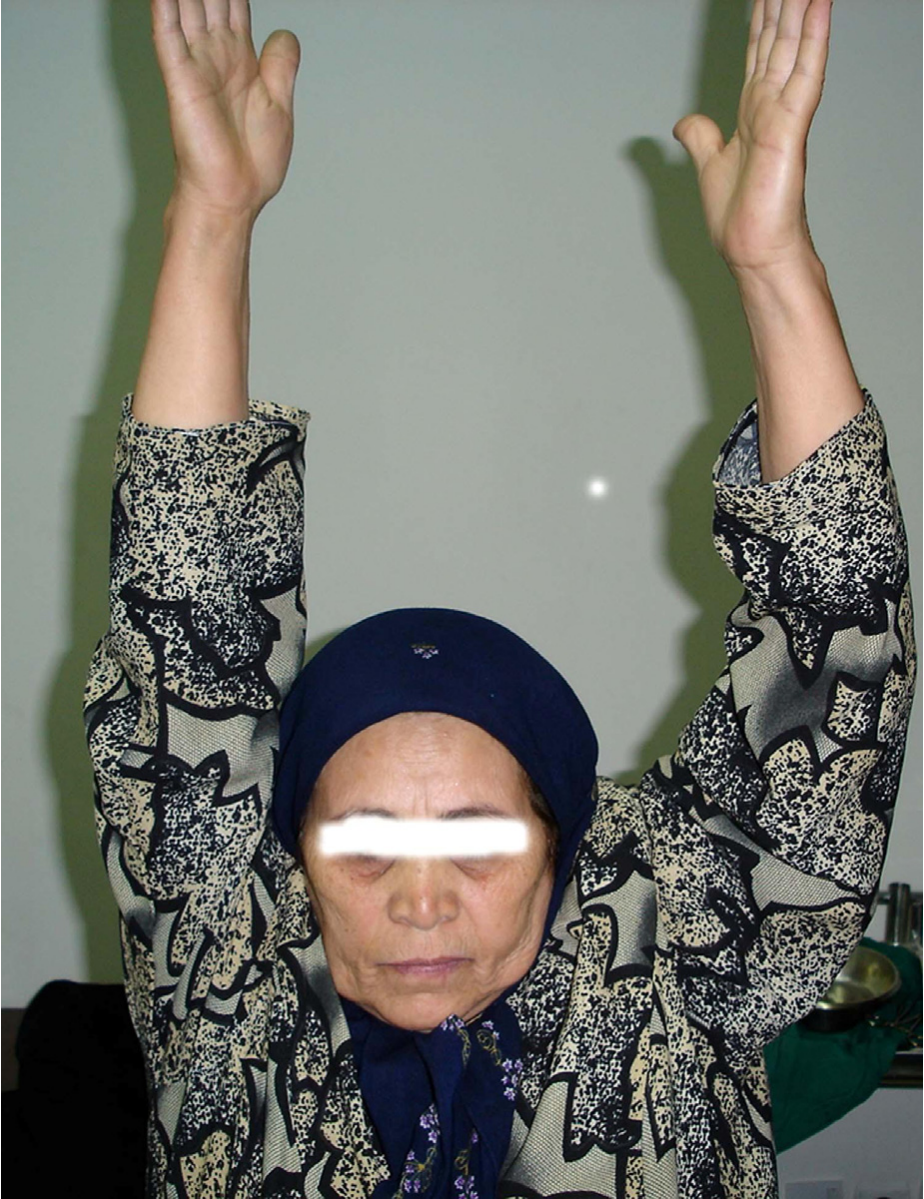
CT scan of 5 week old anterior shoulder dislocation in a 65 year old woman And Forward flexion after 1 year.
